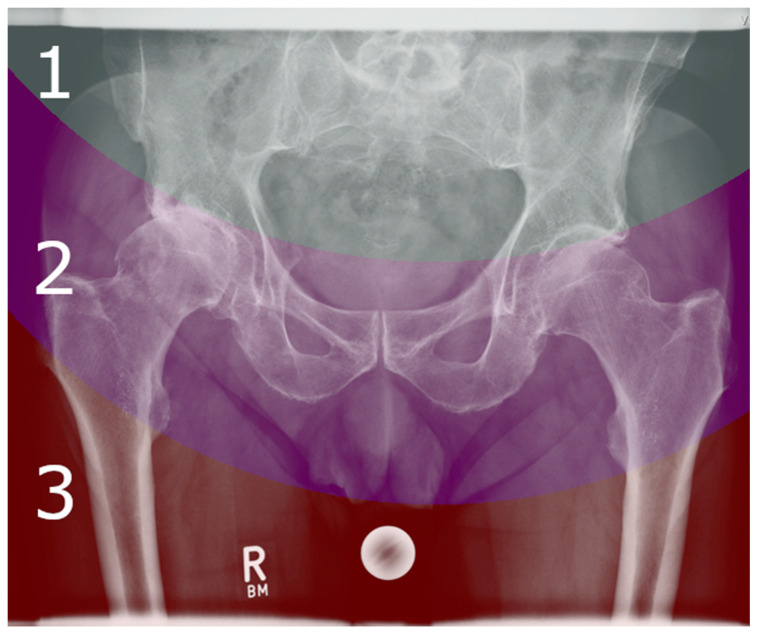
Boundaries when determining our new index: radiograph of the hip, centered on the symphysis. Grading was performed on the left hip with fewer signs of osteoarthritis. If no contour is observable, as in this example, grade 0 is given. A visible contour not projecting on the femur (green) is considered as grade 1. If the fat flap projects on the femur between the head and the lesser trochanter (purple), this results in grade 2. A projection below the lesser trochanter (red) is graded as 3.
